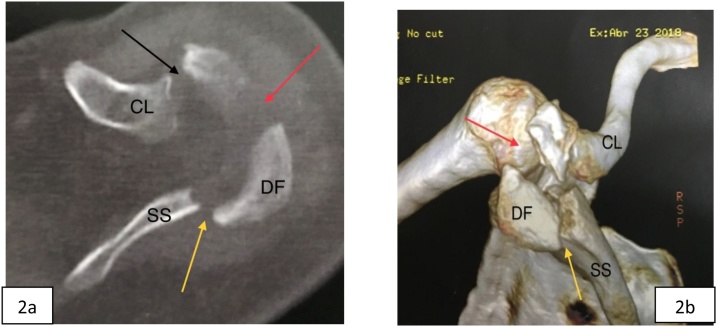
Left shoulder CT (a) with 3D reconstruction (b). yellow arrow: displaced fracture of lateral angle of the scapular spine; red arrow: displaced acromion fracture; black arrow: acromioclavicular joint; CL- clavicle; DF- displaced fragment; SS- spine of the scapula.
